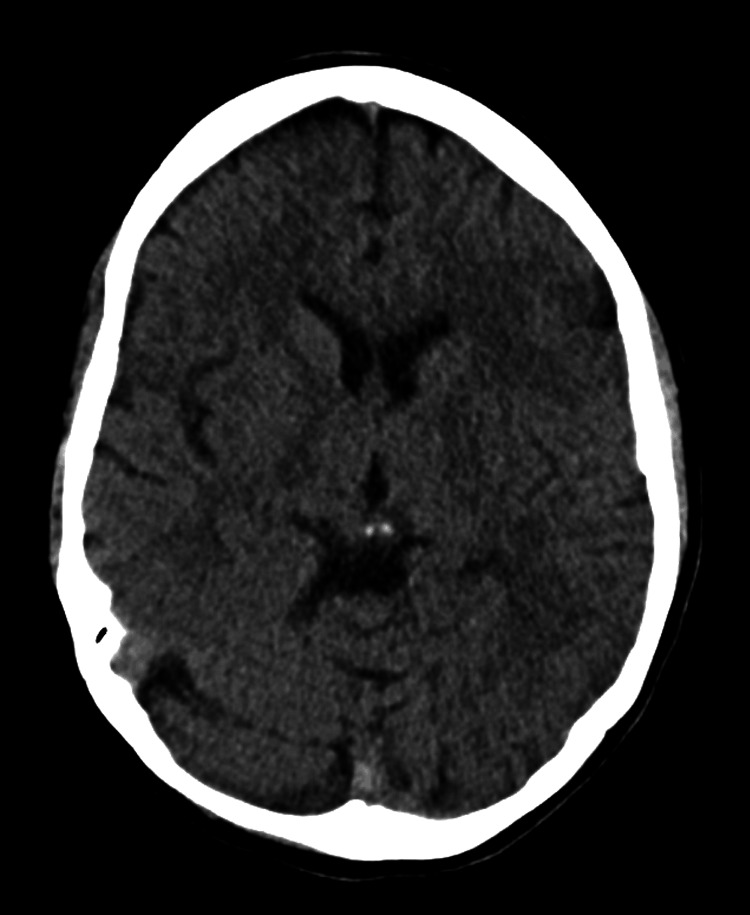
Computed tomography of the head suggesting a normal imaging study
